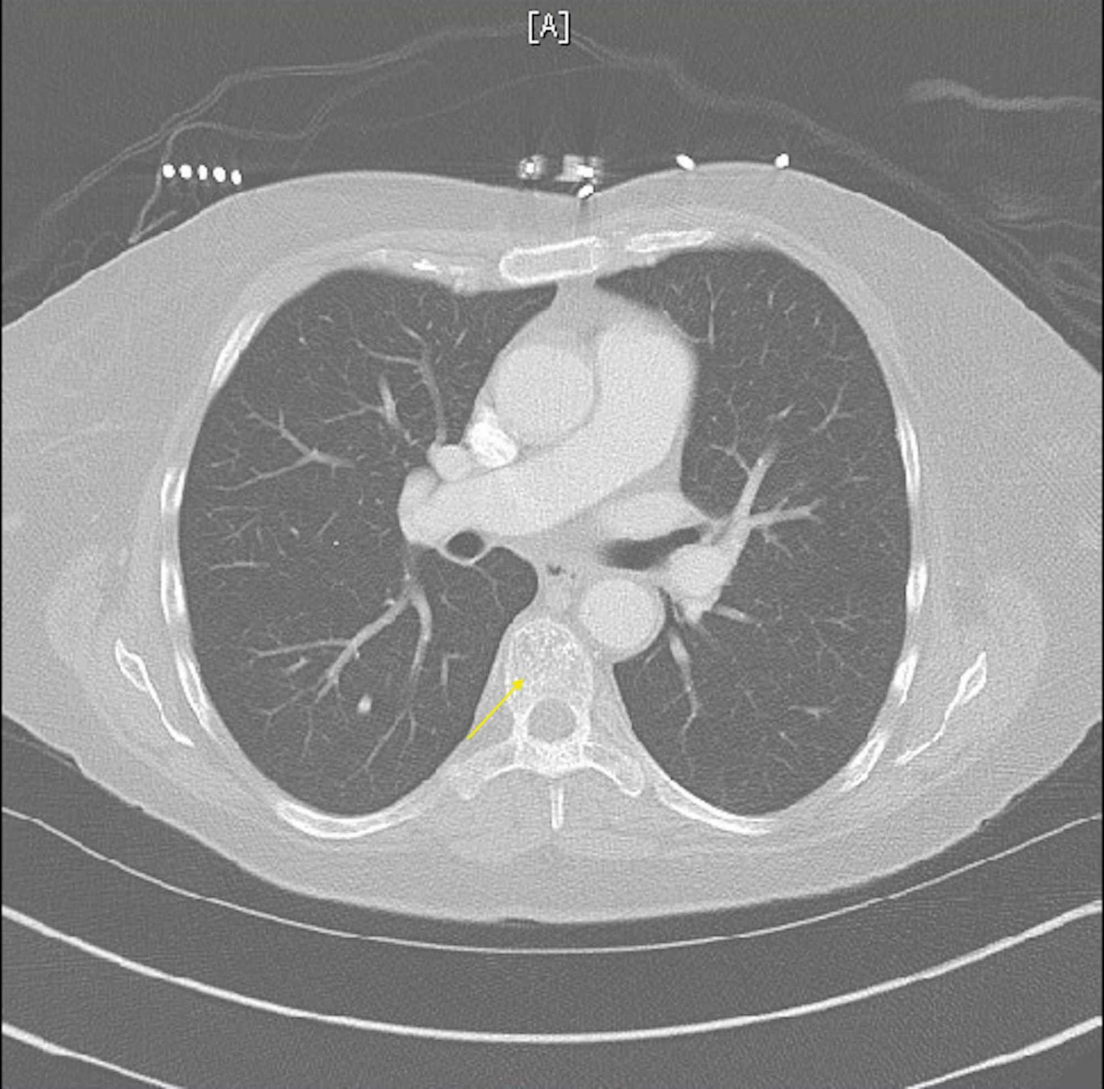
CT of the chest without contrast Diffuse lytic and sclerotic bone lesions can be seen.
